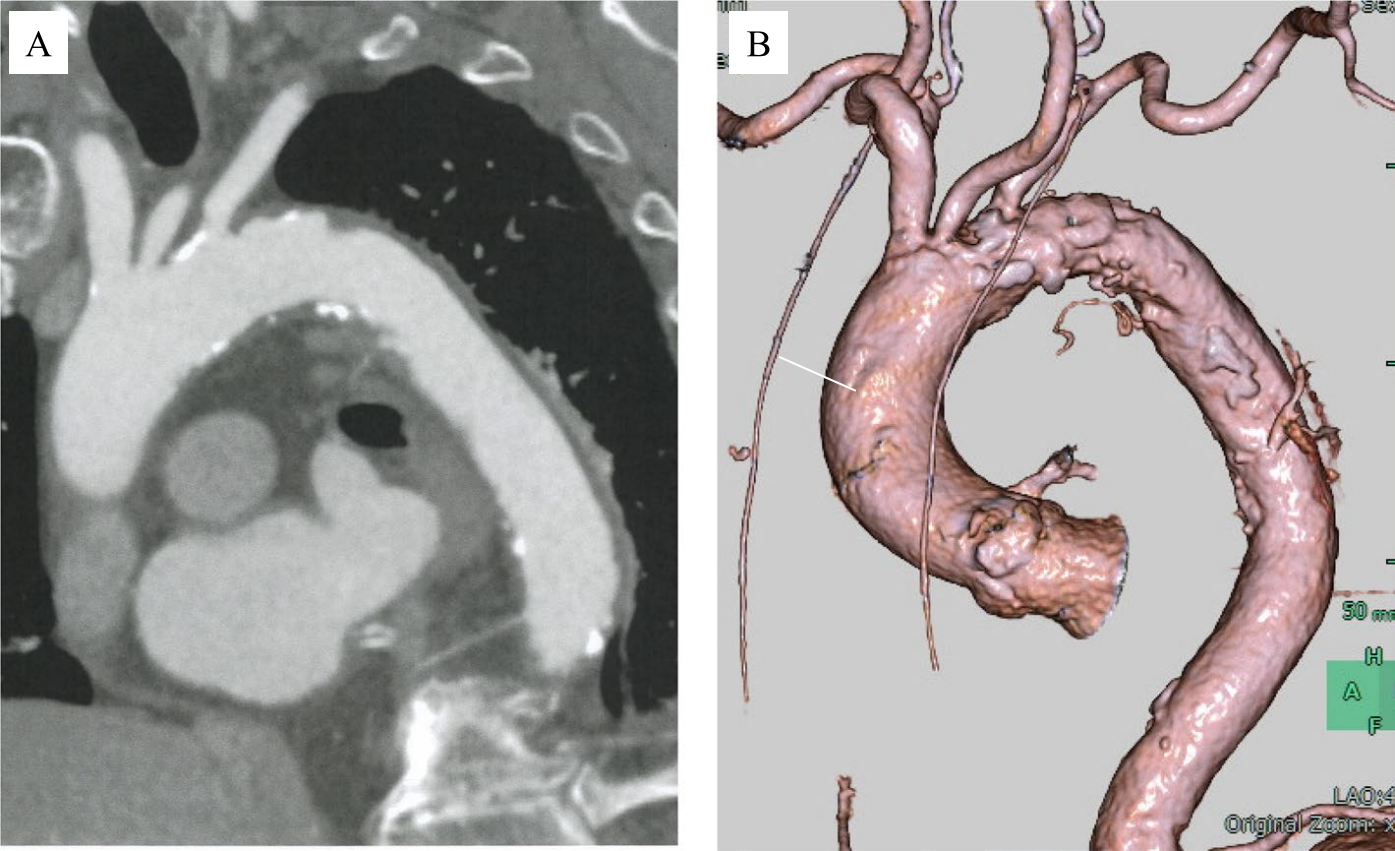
Preoperative CT. A A shaggy aorta from the aortic arch to the descending aorta. B Significant tortuosity of the right subclavian artery and severe calcification of the left subclavian artery
